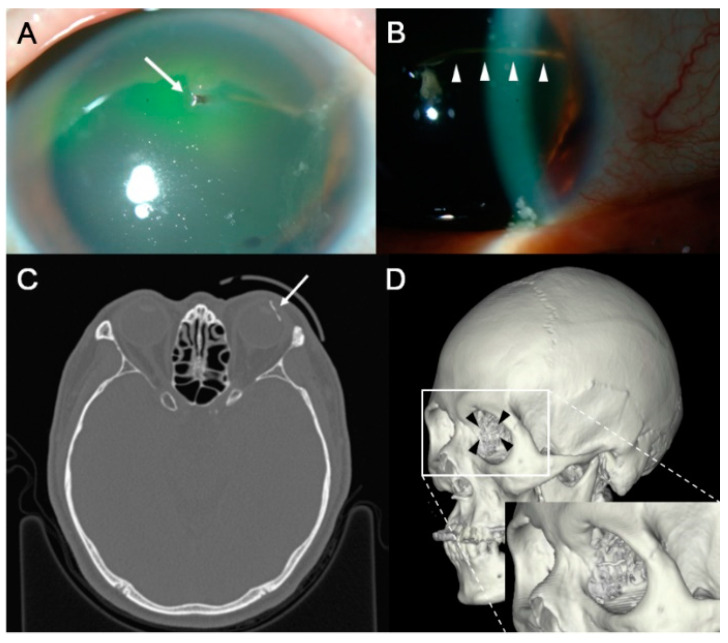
Anterior segment photograph and CT scan of the eye of a 60-year-old male patient who was pierced by a wire during work. (A,B) The distal end of the linear wire has reached the vitreous cavity through iridodialysis and zonule (*white arrowheads*), and the proximal end is exposed outside the cornea (*long white arrow*). On the axial CT scan (C), the direction of the wire (*long white arrow*) is successfully observed, and the 3D reconstructed image (D) shows the shape and position of the wire (*black arrowheads*), which is more remarkable on magnified images (*white box*).
